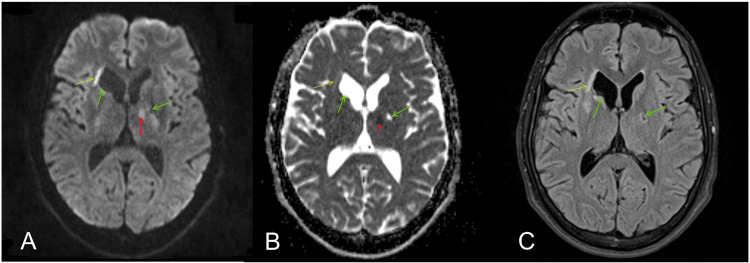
MRI brain scan axial plane sequences DWI (A), ADC (B), and T2-weighted FLAIR (C) showing restricted diffusion of an acute infarction at the left anterior thalamus (red arrows), subacute infarction at the periventricular region of the anterior horn of the right lateral ventricle (yellow arrows), and lesions at the left anterior lateral thalamus and right head of the caudate nucleus that represents the gliosis of chronic infarctions (green arrows). MRI: magnetic resonance imaging; DWI: diffusion-weighted imaging; ADC: apparent diffusion coefficient; FLAIR: fluid-attenuated inversion recovery
